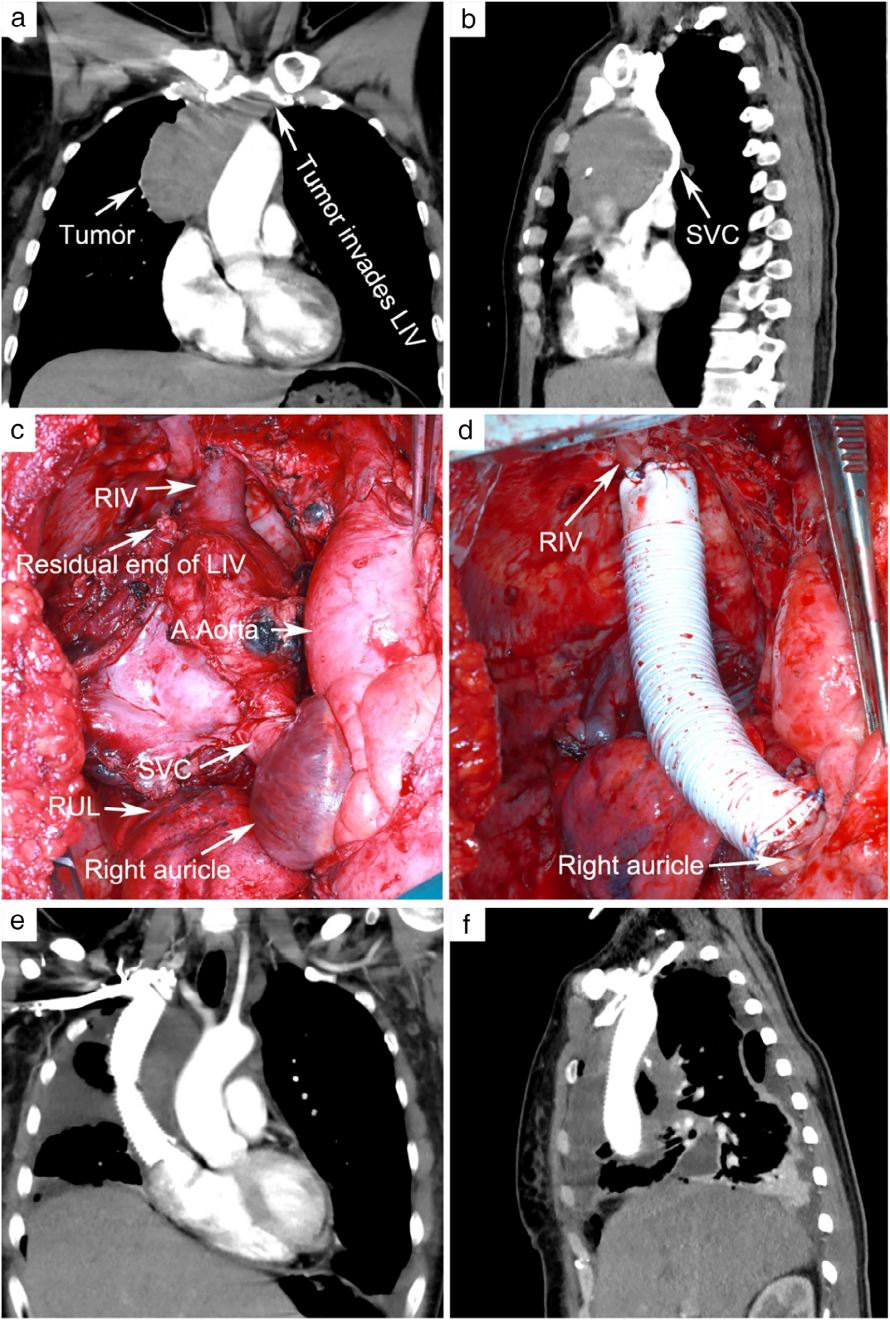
(a and b) Preoperative enhanced CT scan showed the tumor had invaded the left innominate vein and superior vena cava. Tumor thrombus was visible in the superior vena cava. (c) The tumor invaded most of the left innominate vein and the lower part of the right innominate vein to the superior vena cava 1 cm above the sinus node. (d) The artificial blood vessel was anastomosed to the right innominate vein and the right atrium. (e and f) Enhanced CT examination 10 days after surgery showed the unobstructed blood flow in the artificial vessel. LIV, left innominate vein; SVC, superior vena cava; RLV, right innominate vein; RUL, right upper lobe.
